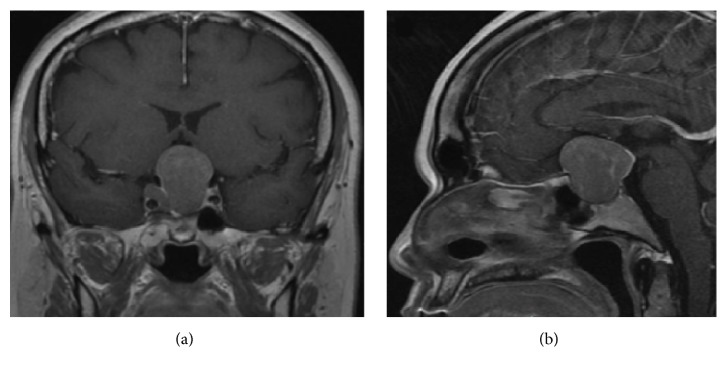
Coronal and sagittal MRI T1 sequence with gadolinium. Intra- and suprasellar tumor with tumor growth related to previous study.
